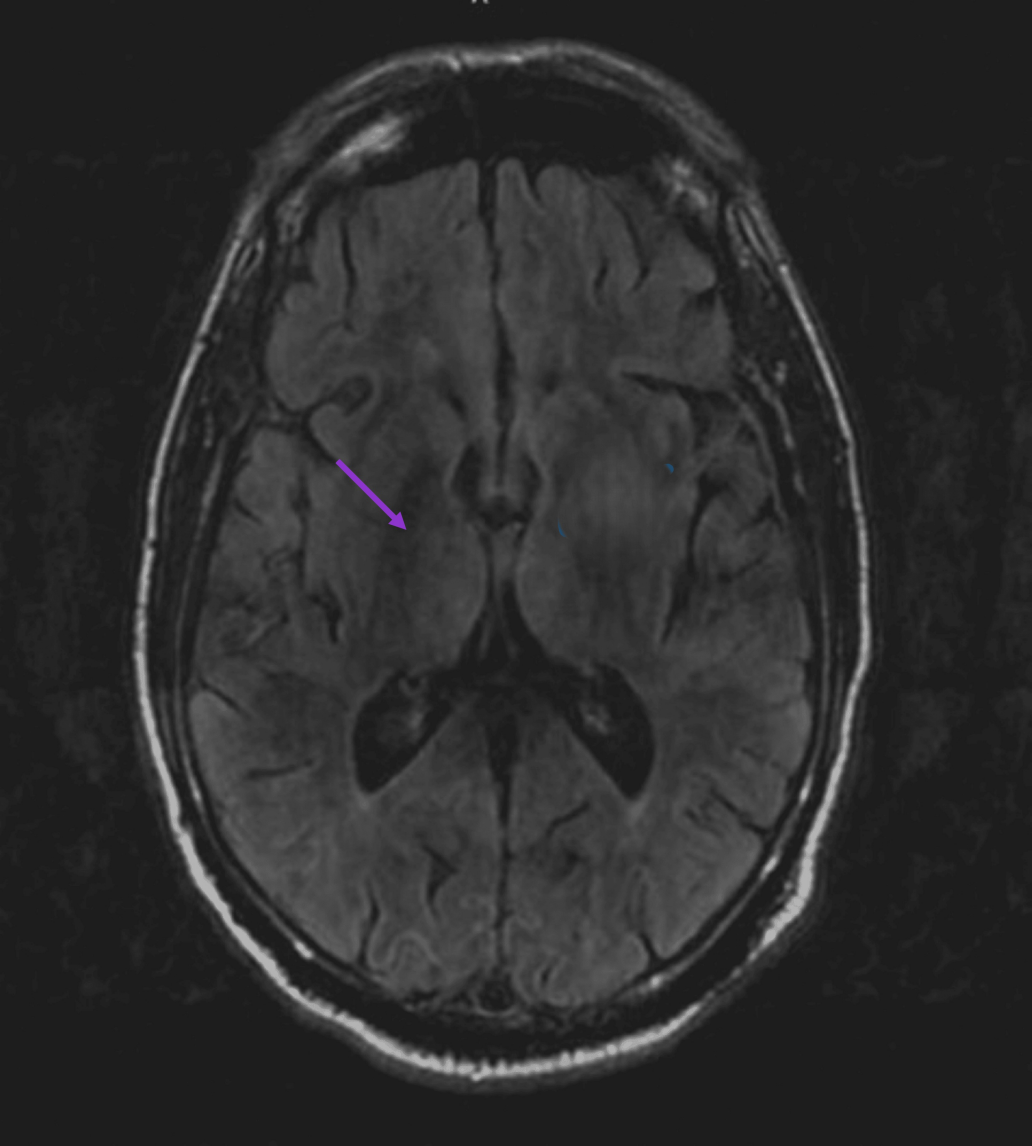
Magnetic resonance imaging of the brain showing symmetrical abnormal fluid-attenuated inversion recovery hyperintensity in the medial bilateral thalami.
